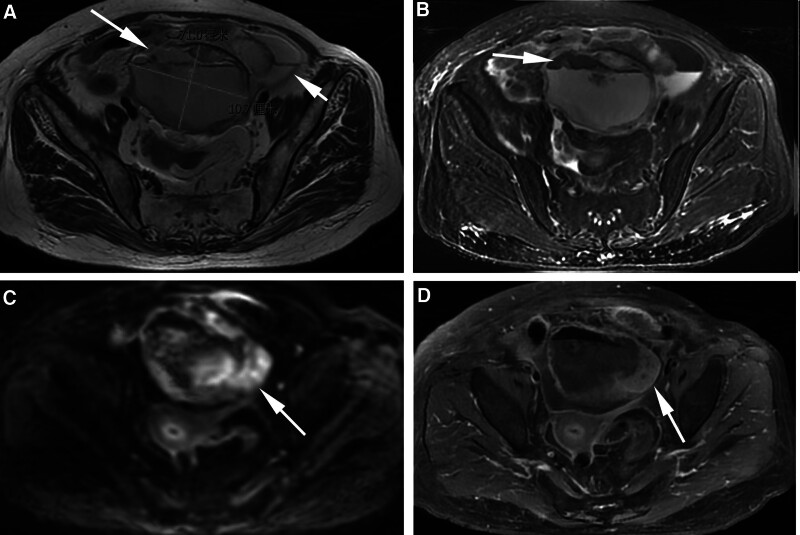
(A) Axial T2-weighted image shows cystic lesion (long arrow) with dimensions of 10.7 × 7.1 cm and ascites (short arrow). (B) Axial T2-weighted image with fat saturation shows low signal intensity of the supernatant fatty layer creating an interface with a high signal intensity aqueous layer (arrow). (C) Axial diffusion-weight (DWI) shows high signal intensity in a section of the tumor wall (arrow). (D) Axial T1-weighted image with fat suppression and contrast enhancement shows which the tumor wall was enhanced (arrow).
